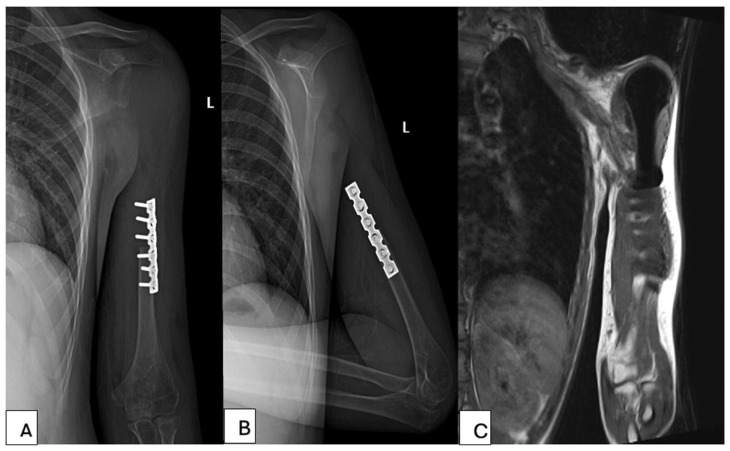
Radiographic follow-up of the patient at 6 months postoperatively. (A,B) show the patient’s anteroposterior and lateral radiographs; (C) shows the postoperative MRI obtained during follow-up evaluation.
